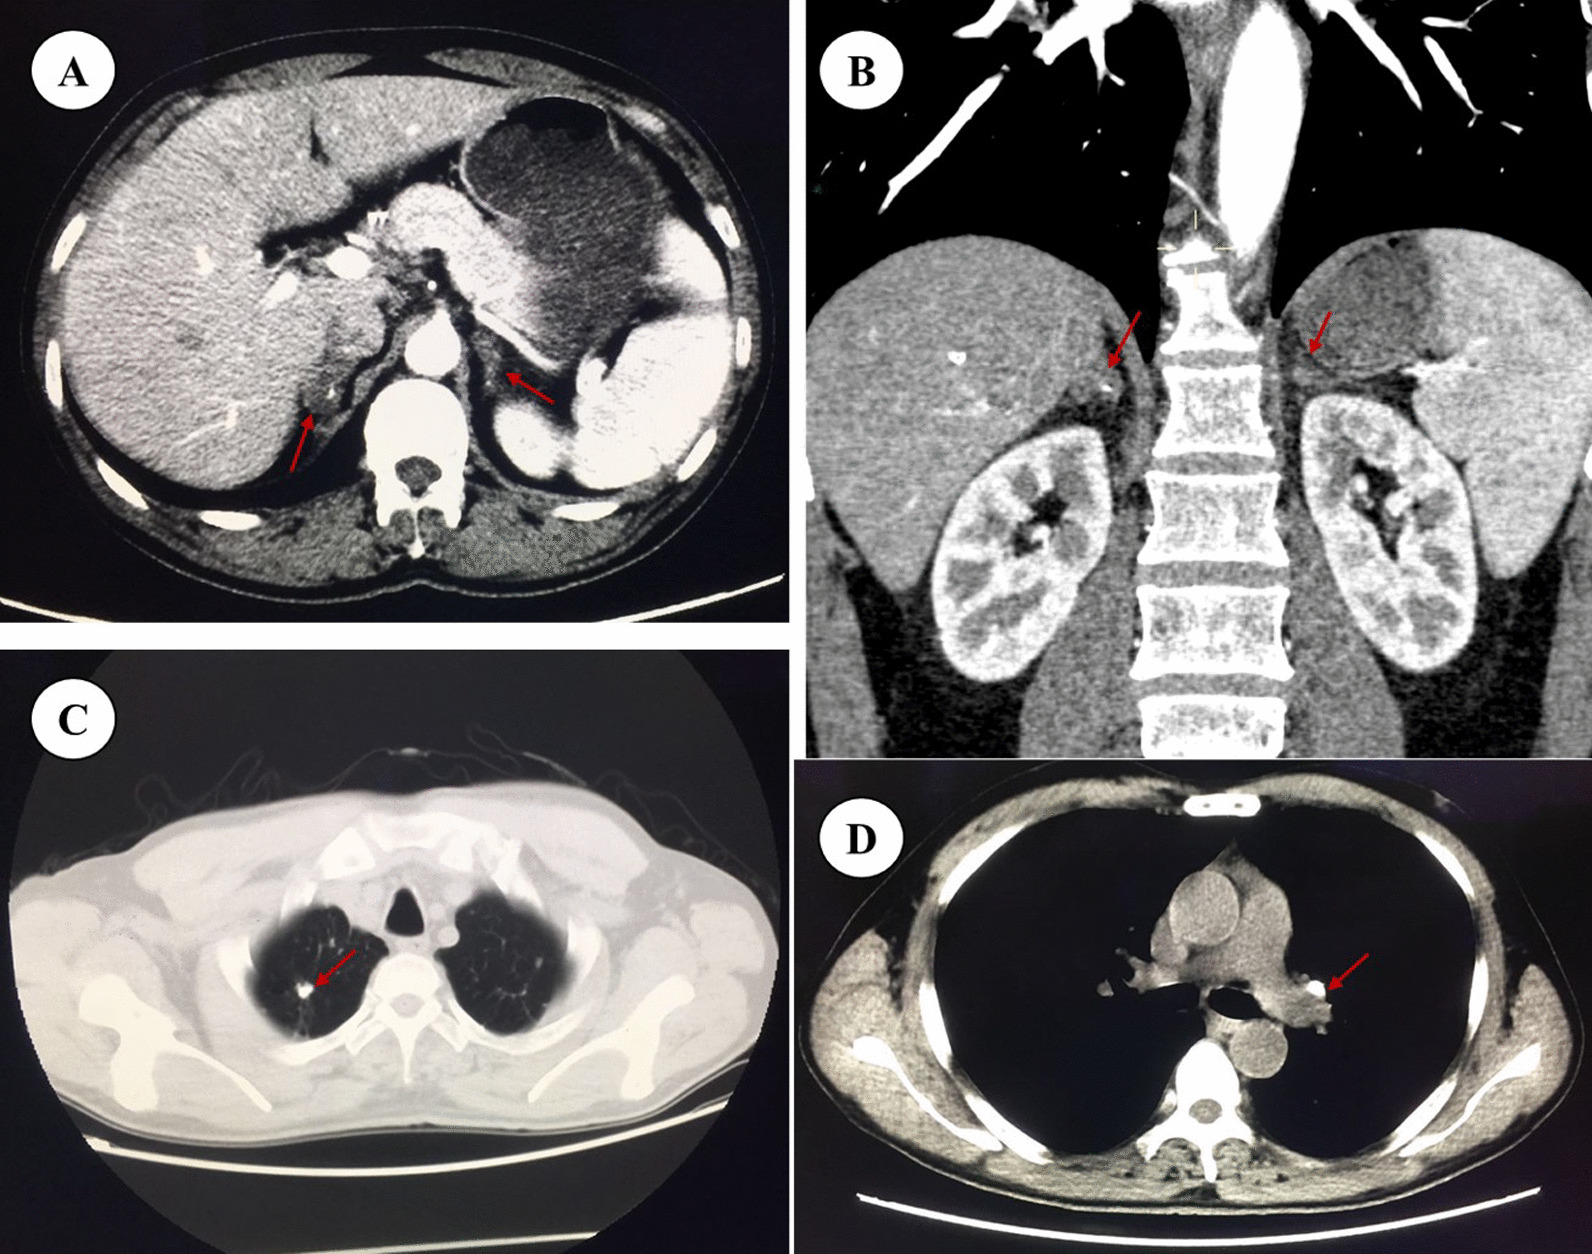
Enhanced CT findings of the chest and abdomen in Addison patients. A, B Visible irregular thickening of the bilateral adrenal glands with a few punctate calcifications (red arrows); C Multiple small nodules, cords, and calcifications in the upper lobes of the lungs (red arrow); D Multiple calcifications in the left hilar and mediastinum lymph nodes (red arrow)
